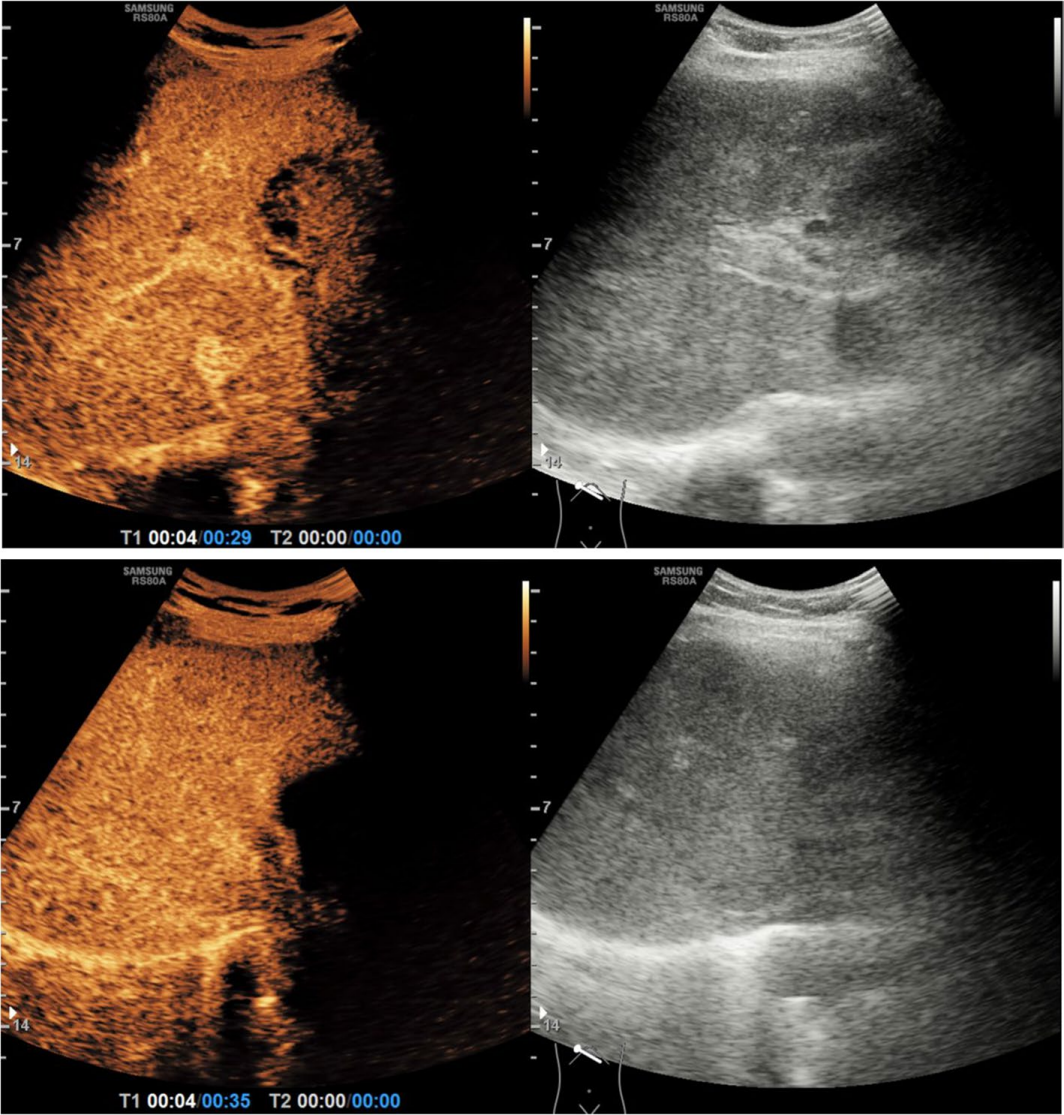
Contrast enhanced ultrasonography after acute liver failure. The echo of liver parenchyma is not uniform, attributed to the high pressure of the hepatic sinusoid. Portal vein perfusion was slightly delayed, there was no hepatic artery-portal vein fistula, and no thrombosis was found in the portal vein
